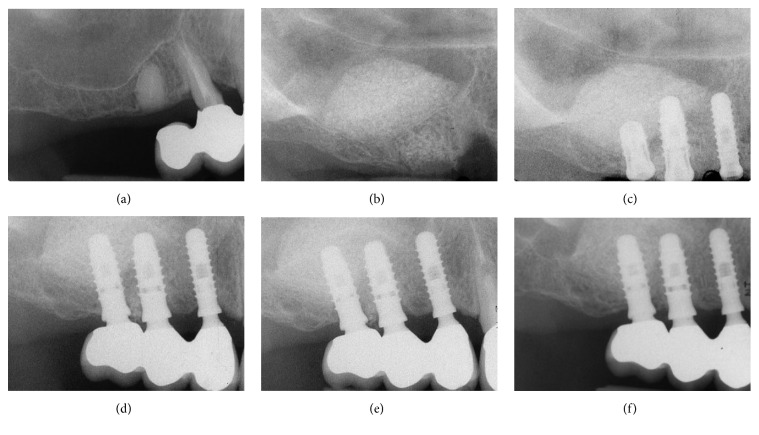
Three implants (#14, #15, and #16) inserted with the lateral window technique. (a) preoperative rx; (b) periapical rx after the sinus augmentation procedure according to Tatum; (c) 6 months later the implants are inserted; (d) radiographic control at the delivery of the final restoration; (e) radiographic control 5 years after implant placement; (f) radiographic control 10 years after implant placement.
